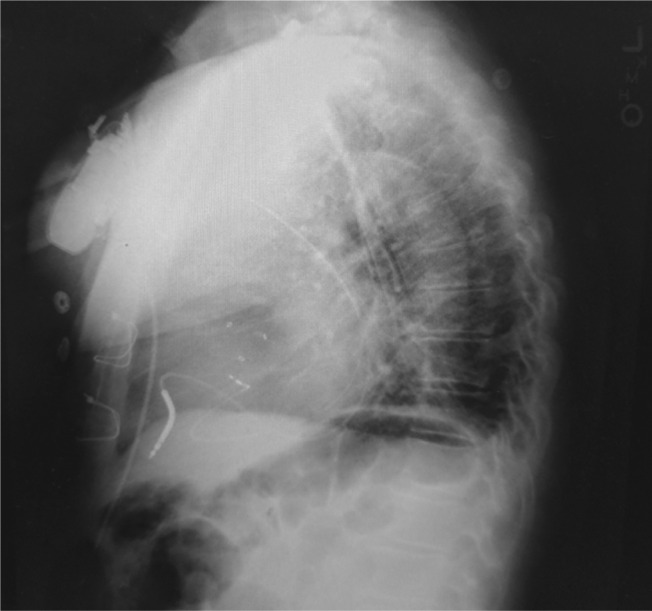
Chest radiograph demonstrating the final His-bundle and ICD lead positions.
